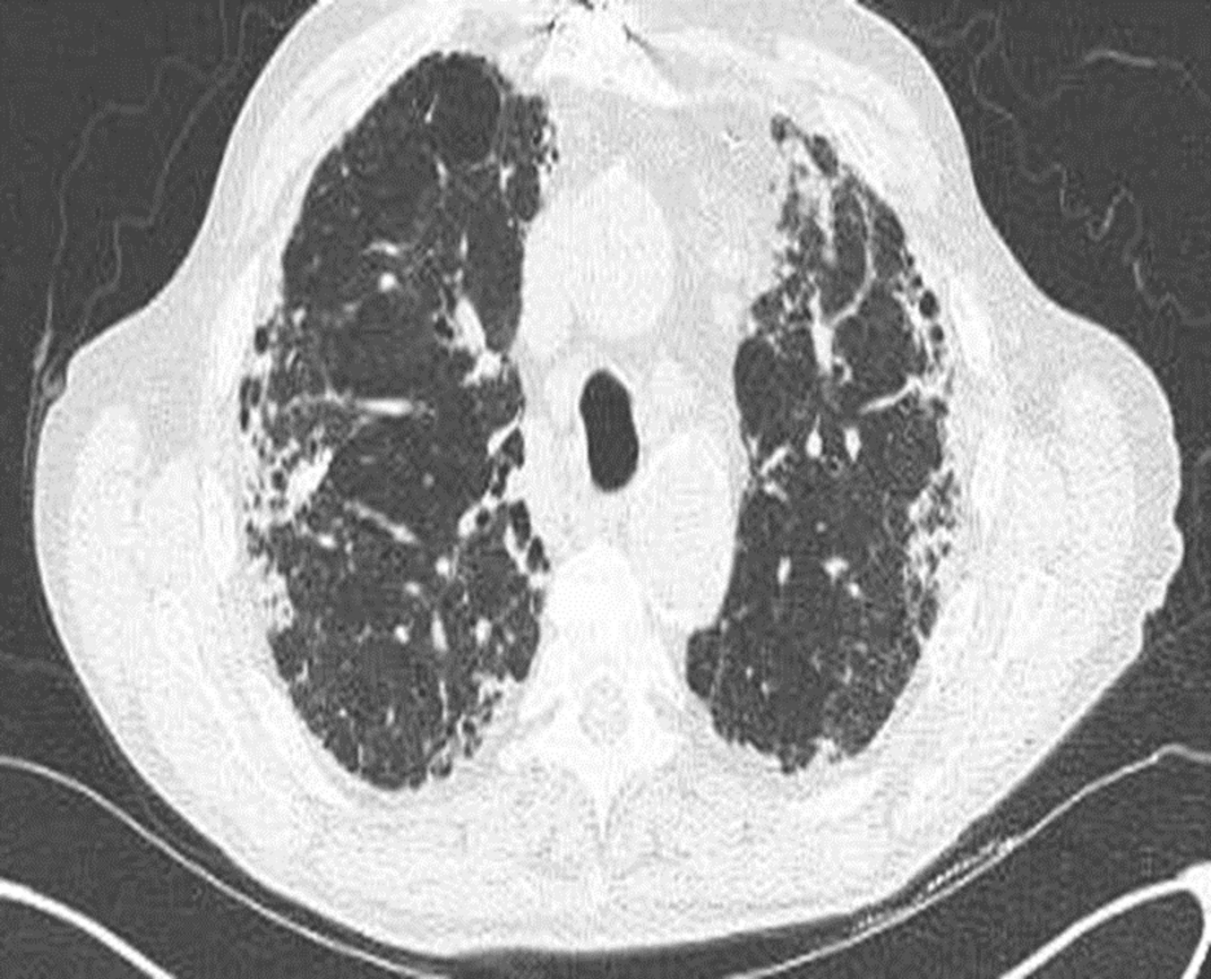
Axial CT chest showing lower lobe predominant fibrotic changes. Axial high-resolution CT image of the chest with no contrast shows reticulation with peripheral distribution involving the subpleural space more predominant in the lower lung field, with traction bronchiectasis, traction bronchioloectasis, honeycombing, architectural distortion, and volume loss diagnostic of usual interstitial pneumonia pattern (sarcoidosis idiopathic pulmonary fibrosis overlap syndrome).
